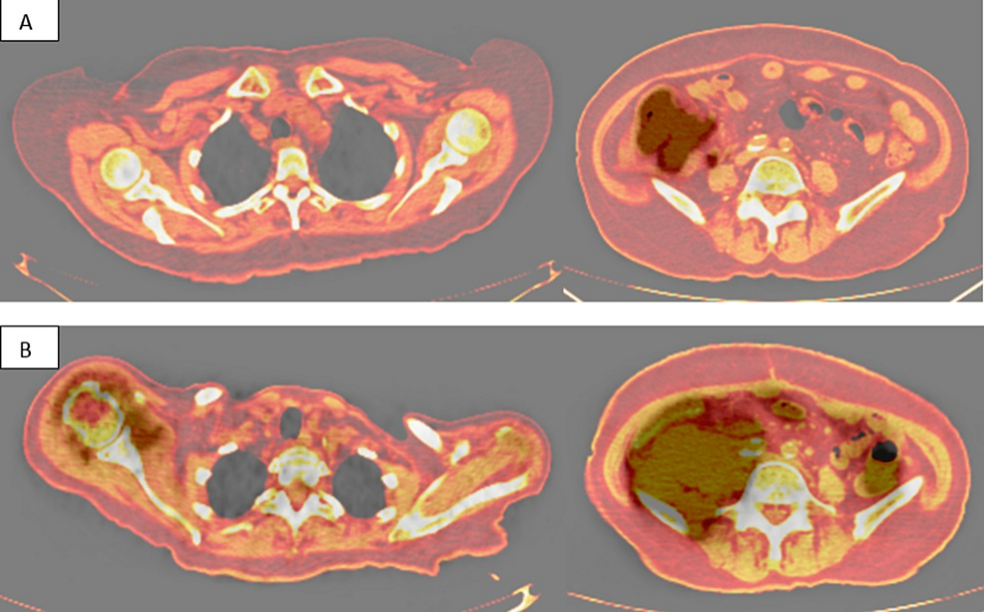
(A) PET scan showing evidence of active lymphoma in the cecum and abdominal and pelvic nodes with no metabolic activity over the right shoulder. (B) Three months later, a PET scan showing FDG hypermetabolism at the right deltoid region associated with FDG-avid active lymphoma in the abdomen and pelvis. PET: positron emission tomography; FDG: fluorodeoxyglucose
